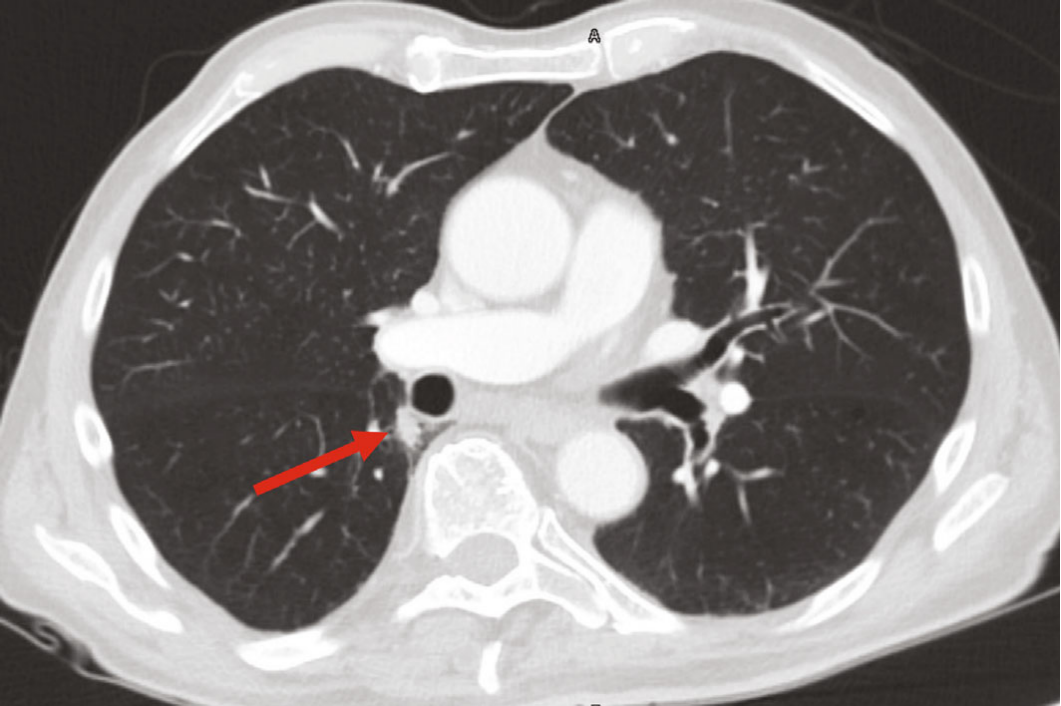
CT of the chest following treatment for peripheral T-cell lymphoma showing a new 1.2 cm nodule in the medial right lower lobe just posterolateral to the distal bronchus intermedius. The nodule appears solid with smooth and spiculated margins and surrounding subtle interstitial thickening. No other obvious abnormalities were noted on CT imaging.
